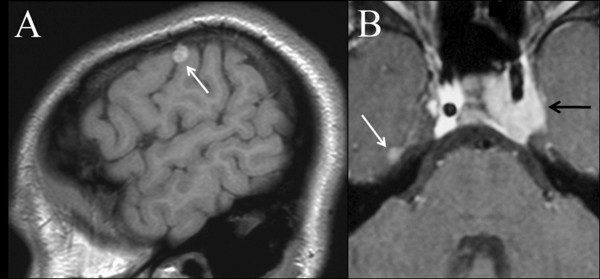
Brain MRI. A) Unenhanced turbo-spin-echo T1-weighted sagittal image documenting the space occupying lesion at the level of the left frontal convexity (white arrow).B) Contrast-enhanced fat-suppressed turbo-spin-echo T1-weighted axial image. MRI documenting the space occupying lesions at the level of the right petrous apex (white arrow) and left cavernous sinus (black arrow).
